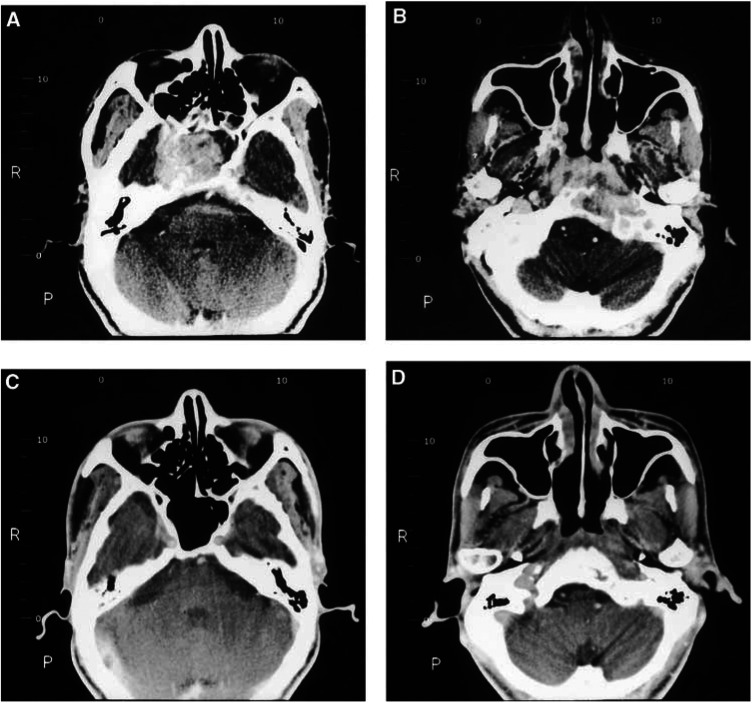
Pretreatment CT scan (A, B) showed a big nasopharyngeal tumour with intracranial invasion in a patient presenting as multiple cranial nerve palsy. The tumour regressed completely with bony regeneration of the destructed skull base after 10-week neoadjuvant chemotherapy (C, D).
